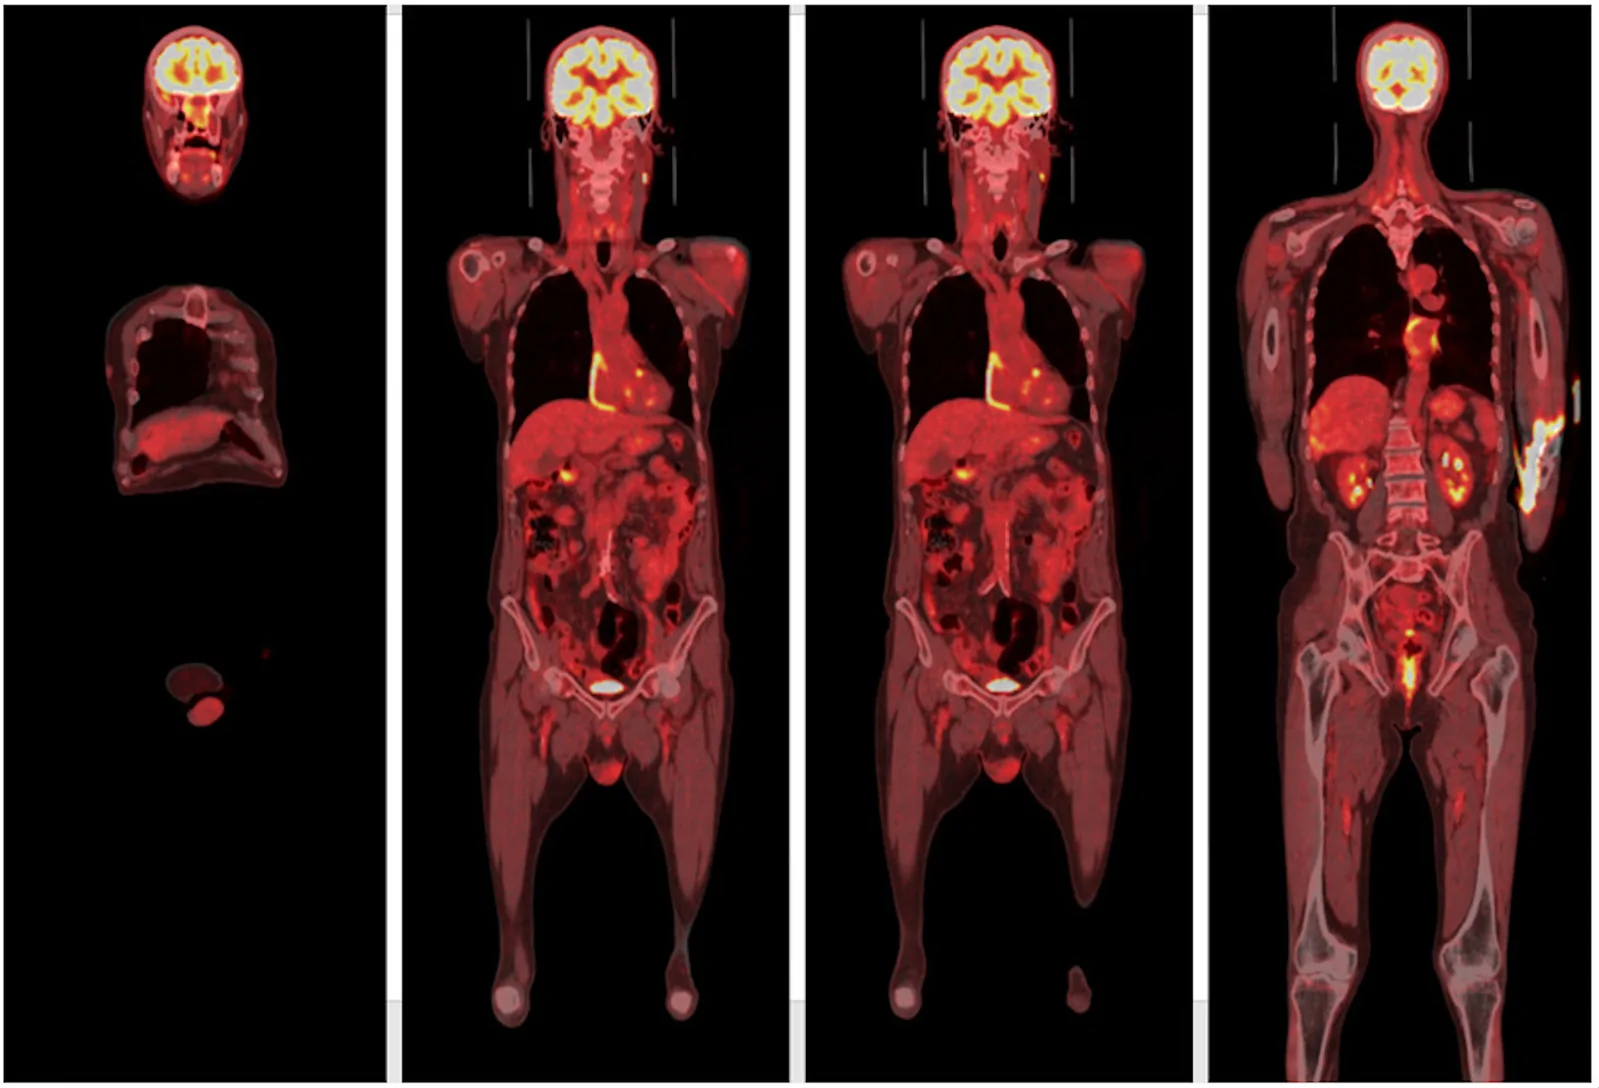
Coronal fusion images of 18F-fluorodeoxyglucose positron emission tomography/computed tomography (18F-FDG PET/CT) scan showing increased 18F-FDG uptake in the sphenoid lesion with ethmoidal extension, in a left retromandibular lymph node, in hepatic segments VI and VII, and nonspecific uptake at the hepatic flexure of the colon.
